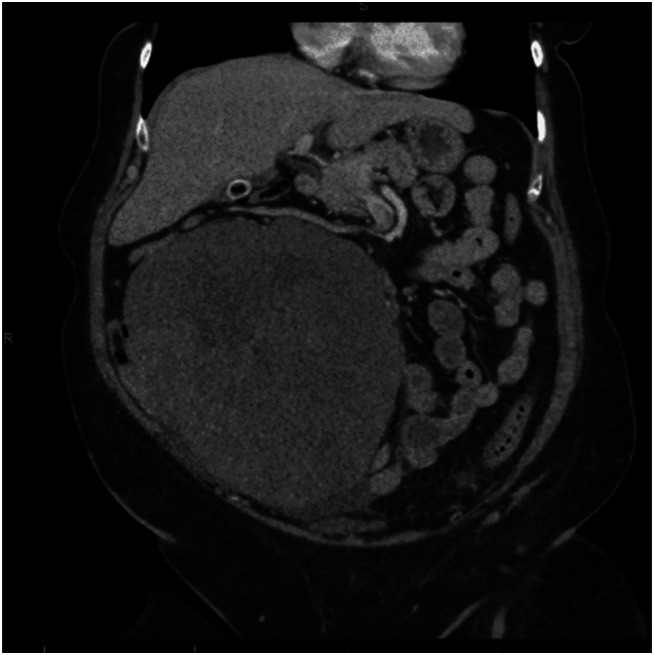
Computed tomography scan shows a 19.7-cm recurrent pelvic mass in 2018.
